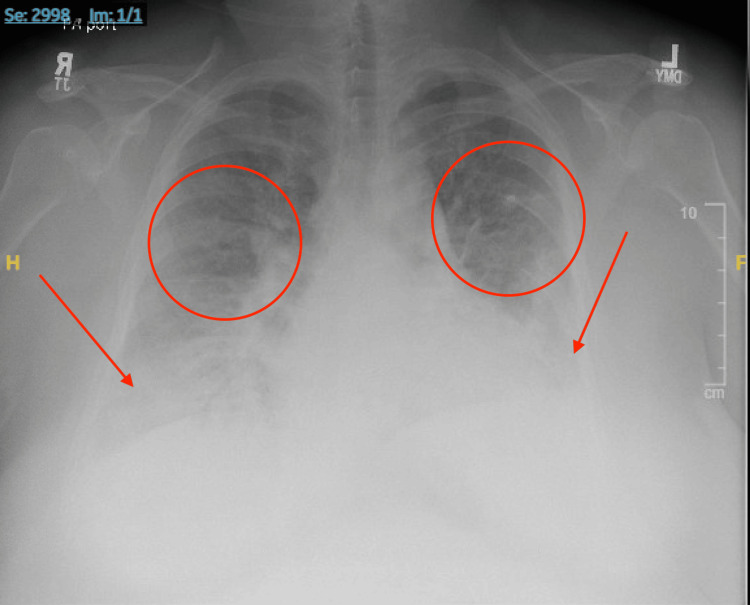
Chest radiograph anterior-posterior view showing perihilar and bibasilar interstitial and airspace opacities (red circles) with small bilateral pleural effusions (red arrows), concerning for pulmonary edema.
